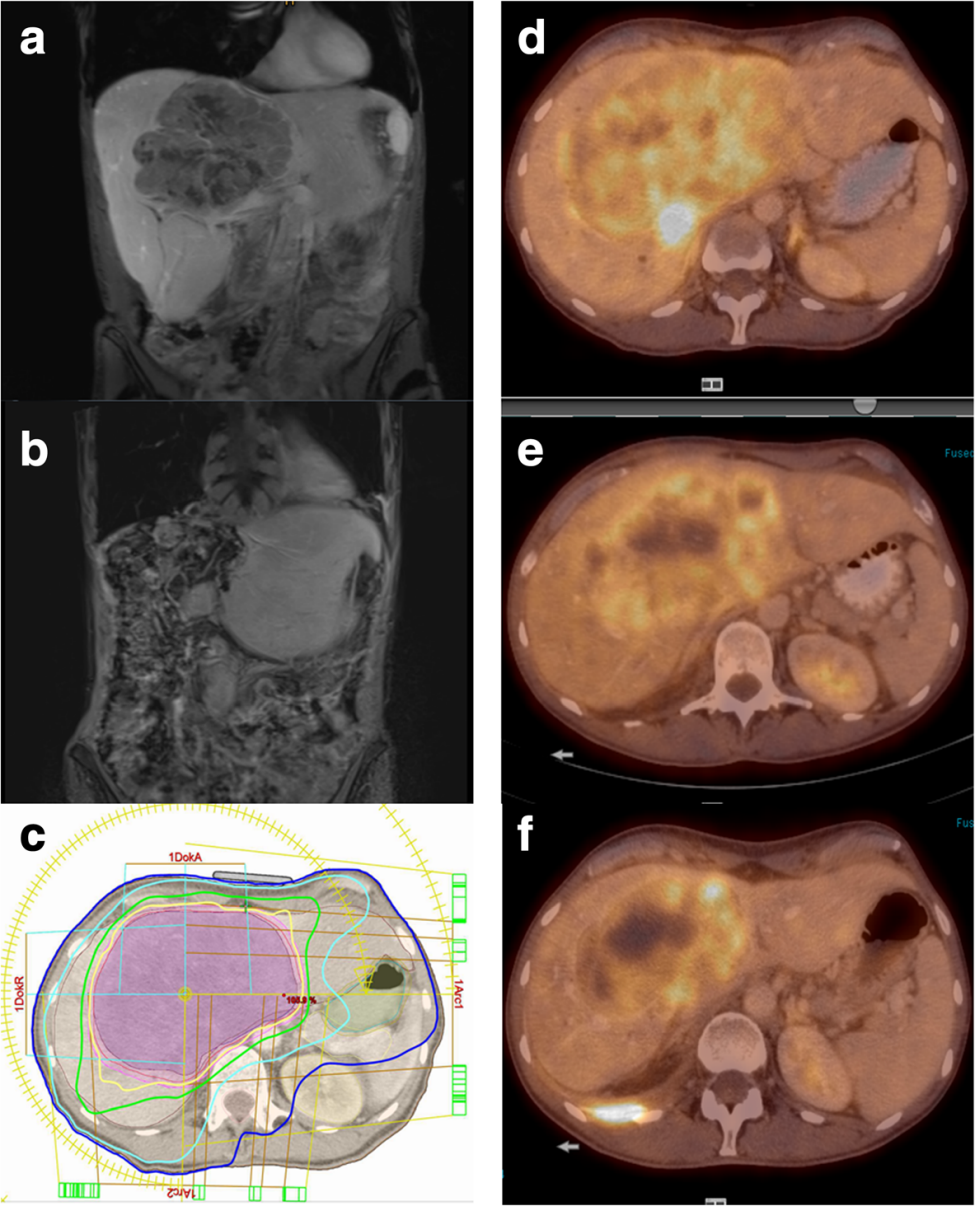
MRI and PET/CT images: a MRI images showing a T1 hypointense, heterogeneous mass (1280 ccm^3^) with peripheral contrast enhancement and washout in segments 1 and 4 to 8 before SBRT, b) MRI images after extended right hepatetctomy, c) Radiotherapy treatment plan (isodose lines: 57 Gy, 48 Gy, 30 Gy, 12 Gy in yellow, green, light blue, and dark blue respectively), d) PET/C before stereotactic body radiotherapy (SBRT), e) PET/CT during SBRT f) PET/CT after SBRT
